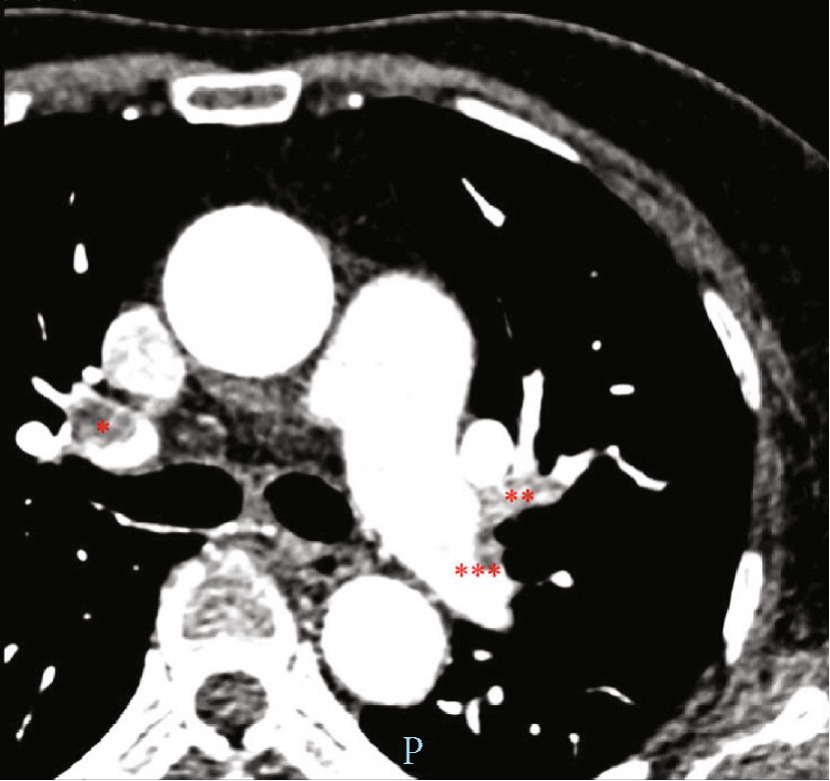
The spiral computed tomography angiogram obtained with highly concentrated contrast material and high flow technique showed multiple pulmonary emboli that subtotally occluded the right upper lobar pulmonary artery (^∗^) and the left lingular artery (^∗∗^). The left lower lobar pulmonary artery was also partially occluded (^∗∗∗^).
